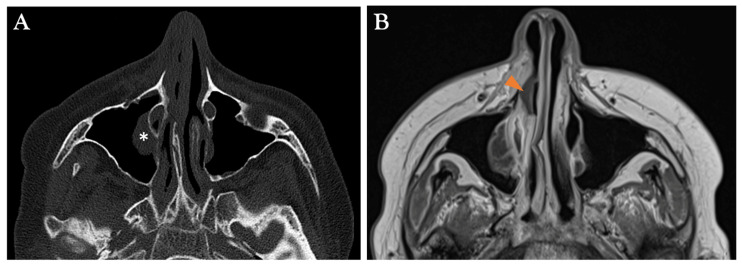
17-year-old male confirmed with right AIFR (*Aspergillus flavus*). (A) CT axial section showing the normal anatomical appearance of the nasal fossa, with a small non-specific mucosal thickening in the right maxillary sinus (asterisk). (B) The corresponding gadolinium-enhanced T1W MRI axial section reveals a non-enhancing area within the right inferior turbinate mucosa (arrowhead), also referred to as the “black turbinate” sign.
